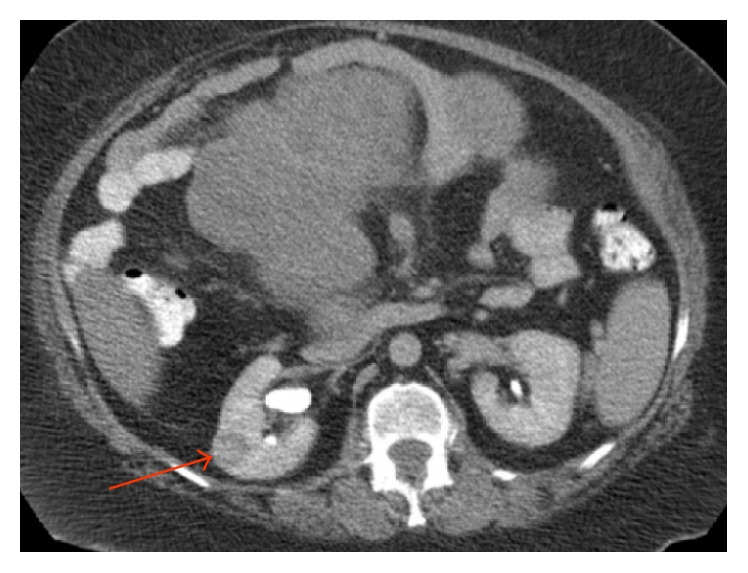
Abdomen CT scan with IV and PO contrast, axial view. Solid mas in the right kidney (red arrow).
